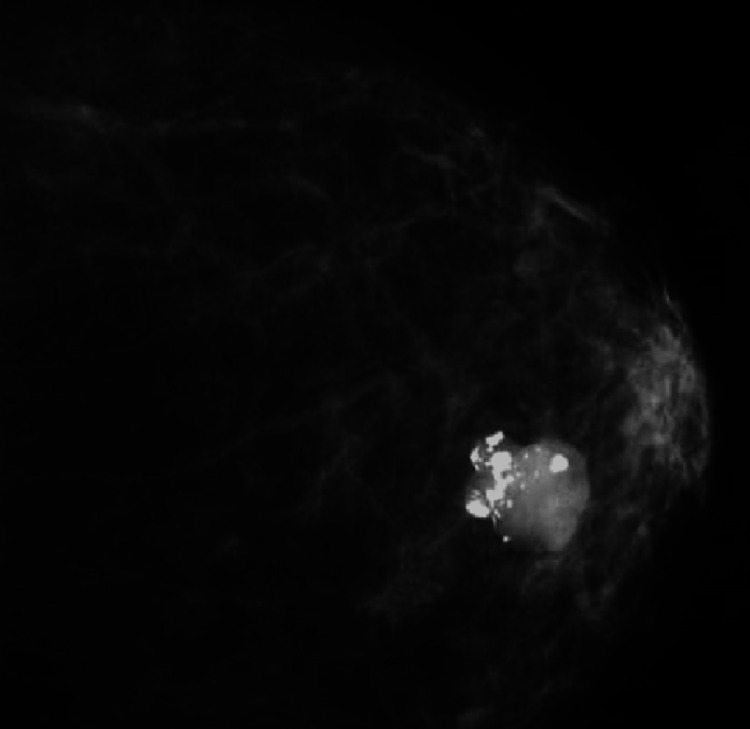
Ultrasound showing a hypoechoic mass in the left breast with lobulated margins and coarse calcifications
